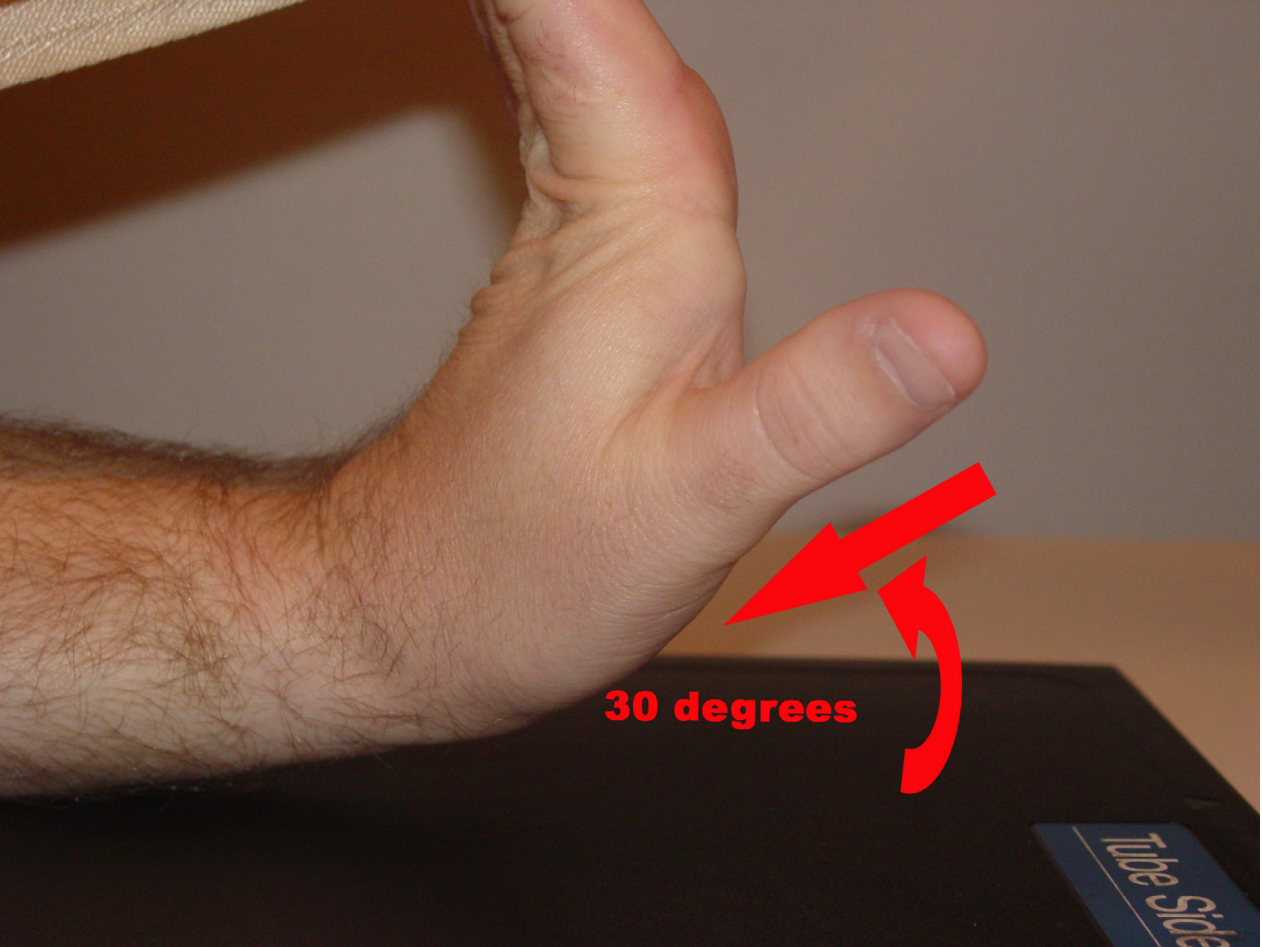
Radiographic position for carpal tunnel view.
